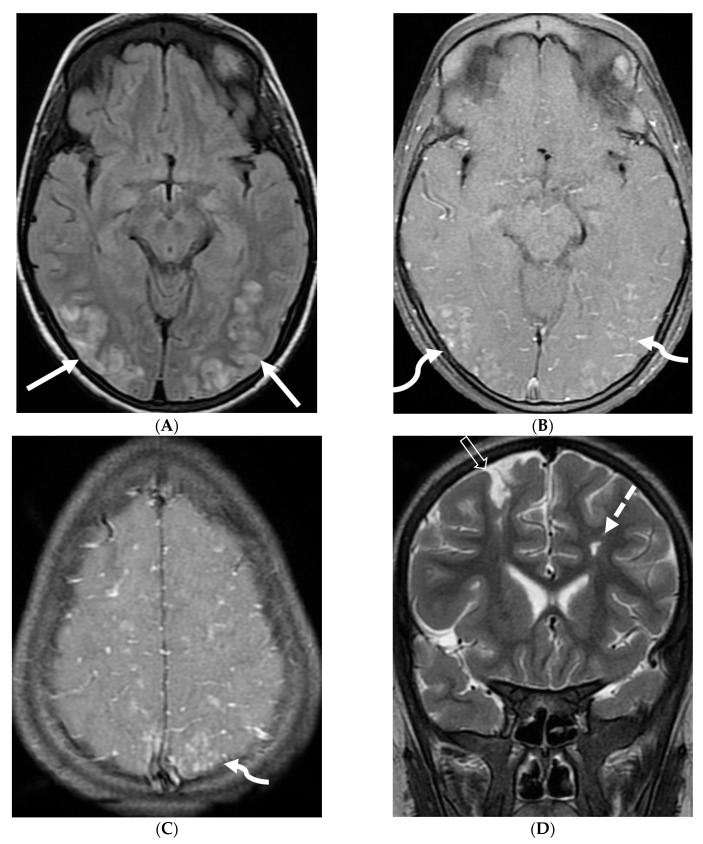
Axial FLAIR (A), post contrast axial T1 (B,C) and coronal T2 (D): 6 year 9-month-old male with sickle cell disease presented with altered mental status, seizure and hypertension. Multiple areas of T2/FLAIR signal hyperintensities are seen in a relatively symmetric distribution involving the bilateral occipital, posterior parietal, high frontal and posterior temporal lobes (arrows). Multiple areas of LME are demonstrated in the involved regions (curved arrows). These findings are characteristic of posterior reversible encephalopathy syndrome (PRES). Areas of encephalomalacia and gliosis involving the deep white matter of bilateral frontal lobes (dashed arrow) and a small area of old cortical infarct involving the right frontal lobe (open arrow), secondary to small vessel disease in a patient with sickle cell disease.
